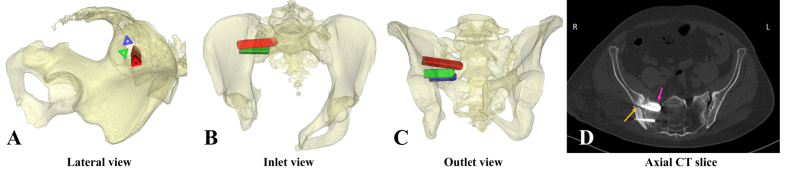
A 3D visualization of the Virtual Surgical Planning (VSP) of the third case in lateral, inlet and outlet view (A–C). It can be seen that the first (red) is placed with a posterior inclination and that the second (green) and third (blue) are placed perpendicular to the true lateral view. The uncommon sacral morphology, hemi-sacralization left and malformed S1 body right, as well as the severe arthritis of both SI joints can be seen. In the axial CT slice (D), a minor breach of the anterior sacrum (highlighted by the orange arrow) and an ilium fracture (pink arrow) are depicted. (For interpretation of the references to color in this figure legend, the reader is referred to the Web version of this article.)
